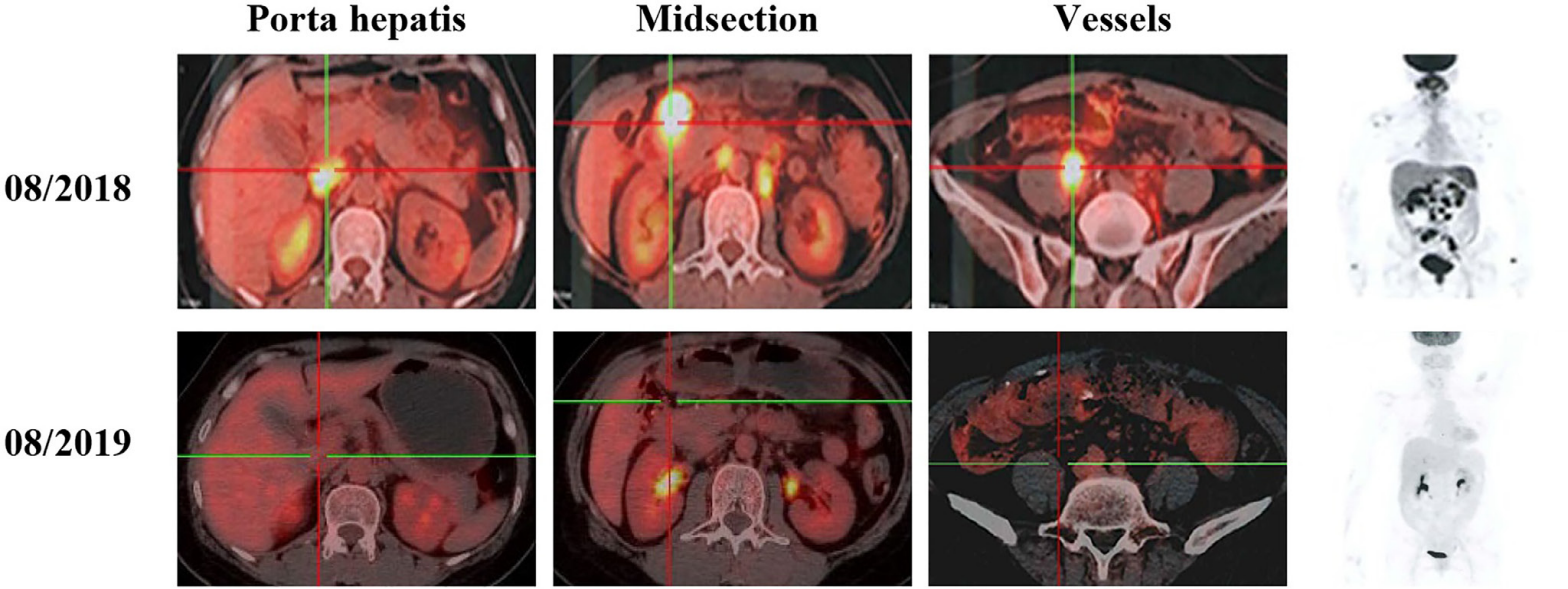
PET-CT scans show the patient’s therapeutic response following a series of treatments, including second-line and third–line therapeutic regimen based on nivolumab.
